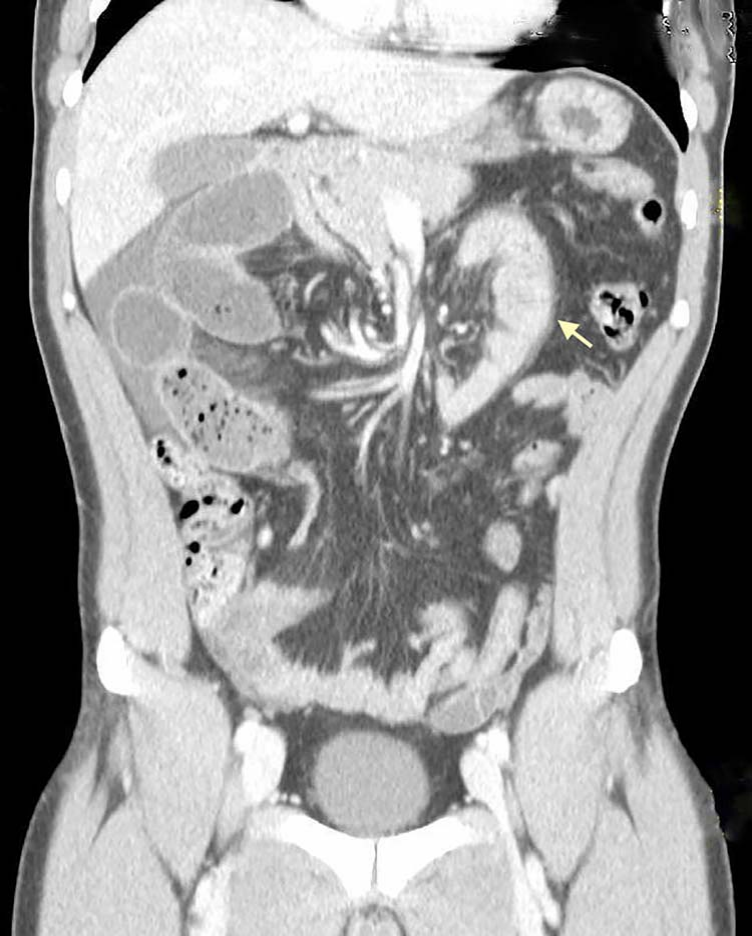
Coronal CT scan shows signs of intestinal malrotation, with alteration of the normal topography of the duodenal arch (arrow).
